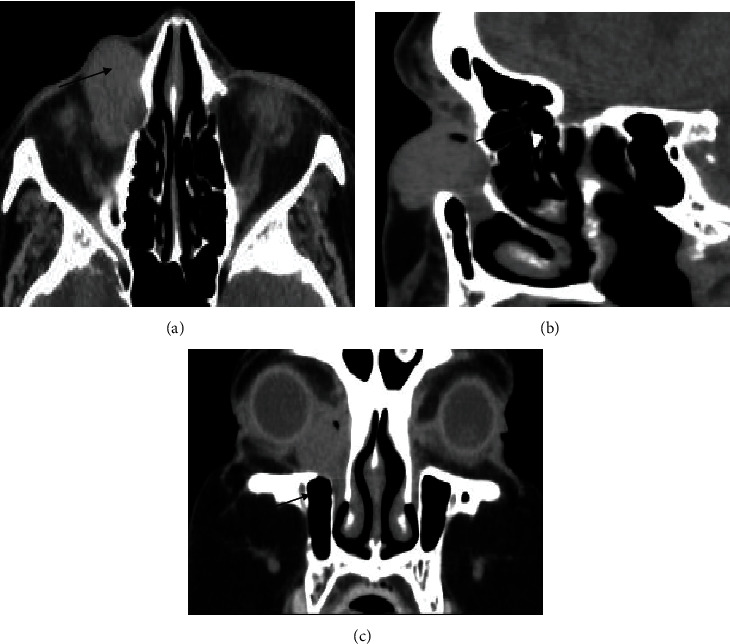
(a–c) Orbital computed tomography demonstrating an oval, solid, well-circumscribed, homogeneous mass extending from the lacrimal sac into dilated duct without any evidence of invasion into adjacent bones. (c) Coronal CT scan before the second excision.
